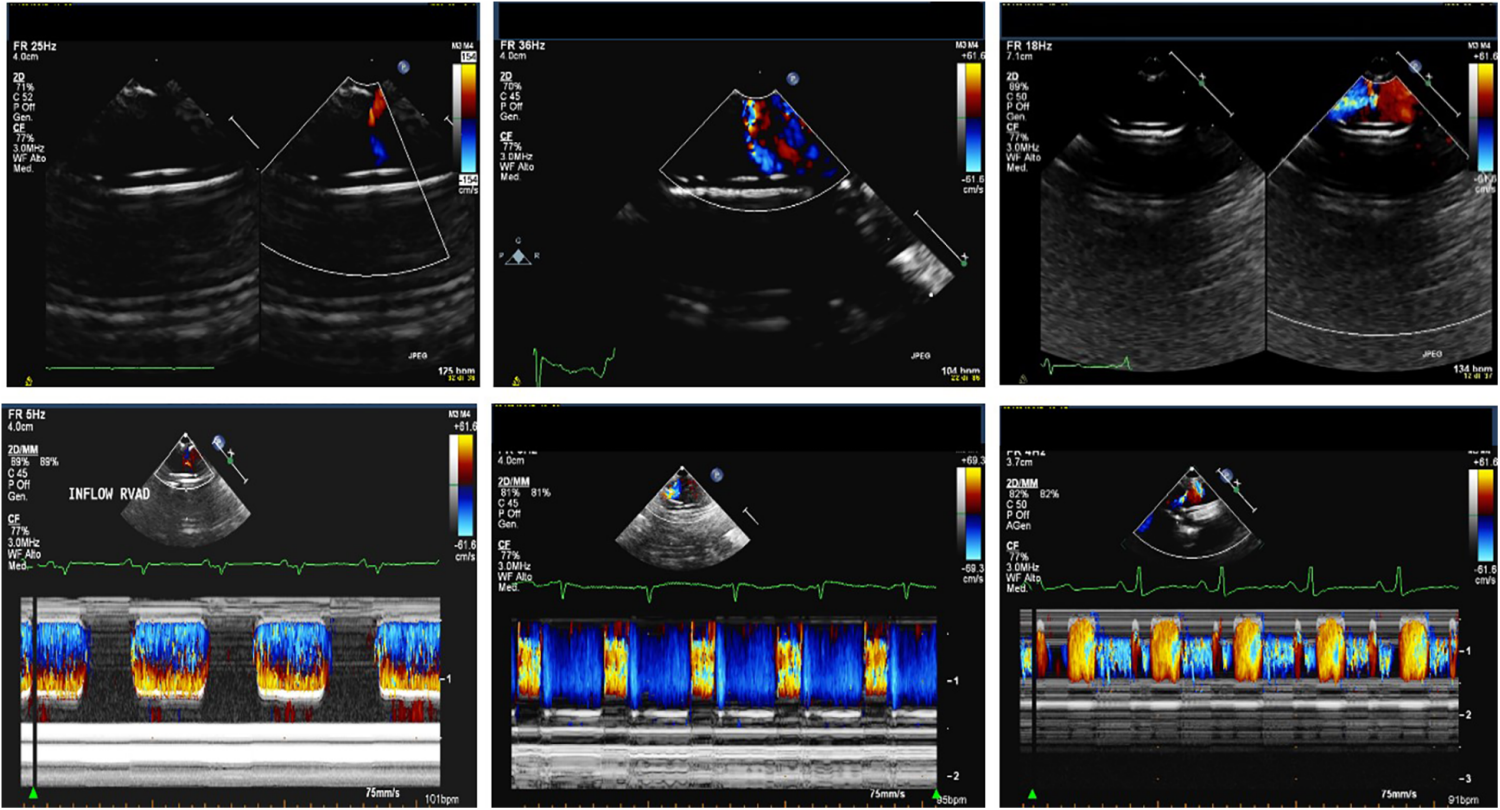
2D color and M-mode color Doppler of no regurgitant Berlin heart valve (left), a Berlin heart valve with a mild regurgitation (middle) and a Berlin heart valve with a moderate regurgitation (right).
